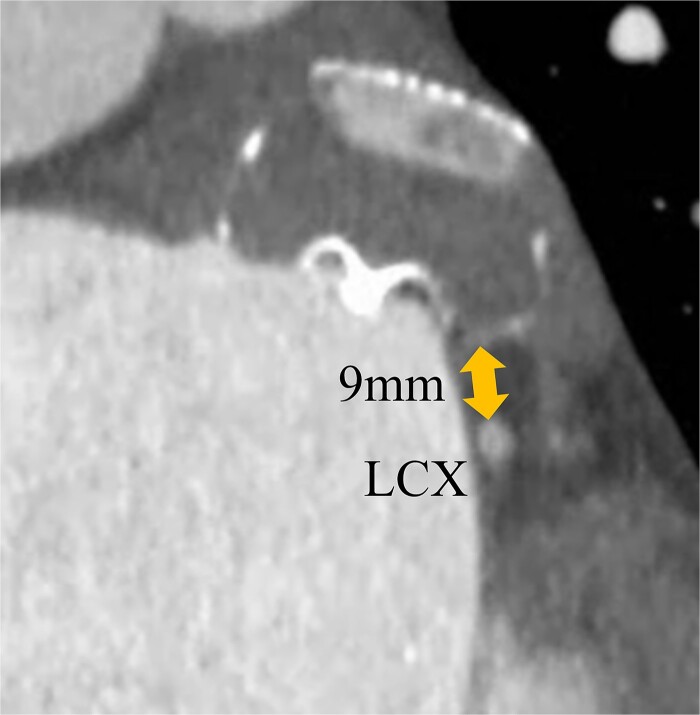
Preoperative enhanced computed tomography imaging showing the distance between the WATCHMAN device and the LCX was ~9 mm.
